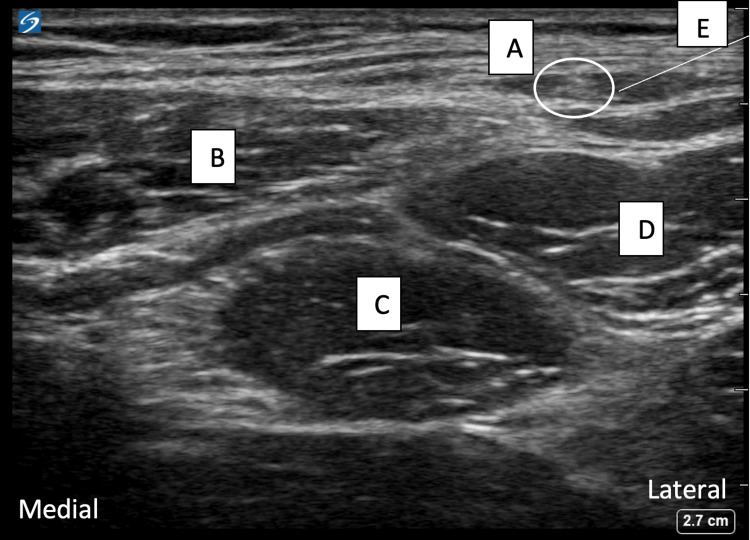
An example of LFCN ultrasound anatomy The image shows the lateral femoral cutaneous nerve (A), sartorius muscle (B), rectus femoris (C), tensor fascia lata (D), and needle trajectory (E). LFCN: Lateral femoral cutaneous block.
